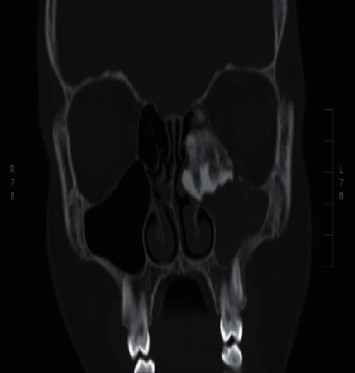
The CT scan of the paranasal sinuses shows a left ethmoid mass.
